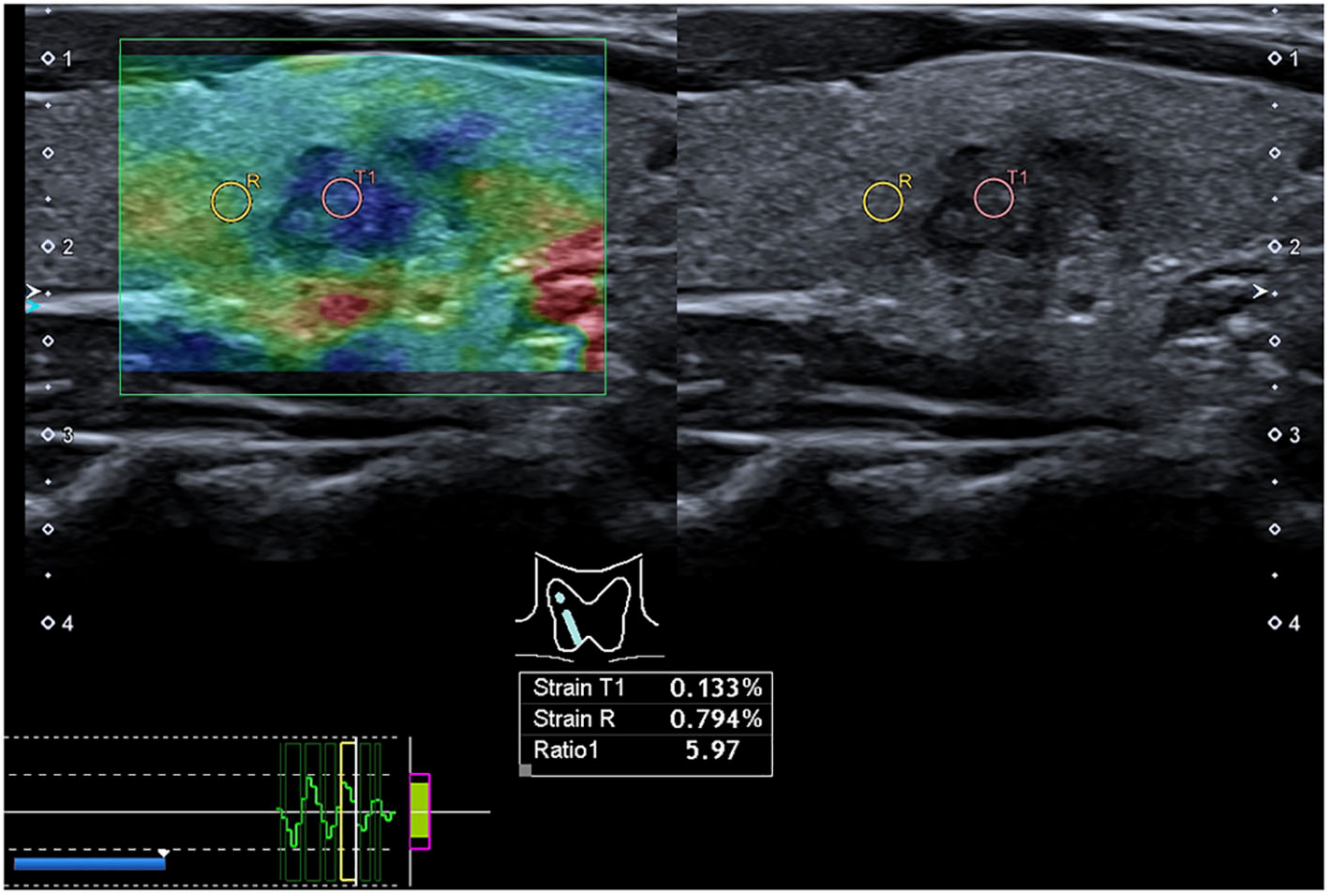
Preoperative strain ultrasound elastography images. Preoperative strain ultrasound elastography of a patient with papillary thyroid cancer in the right lobe of the thyroid. The image on the left shows the hardness distribution of tumor tissue (T1) and surrounding thyroid parenchyma (R) in a green sampling box. The color distribution is from red to blue (blue areas indicate hard areas, and red areas indicate soft areas). The right image shows a gray-scale ultrasound image corresponding to the strain ultrasound elastography image.
